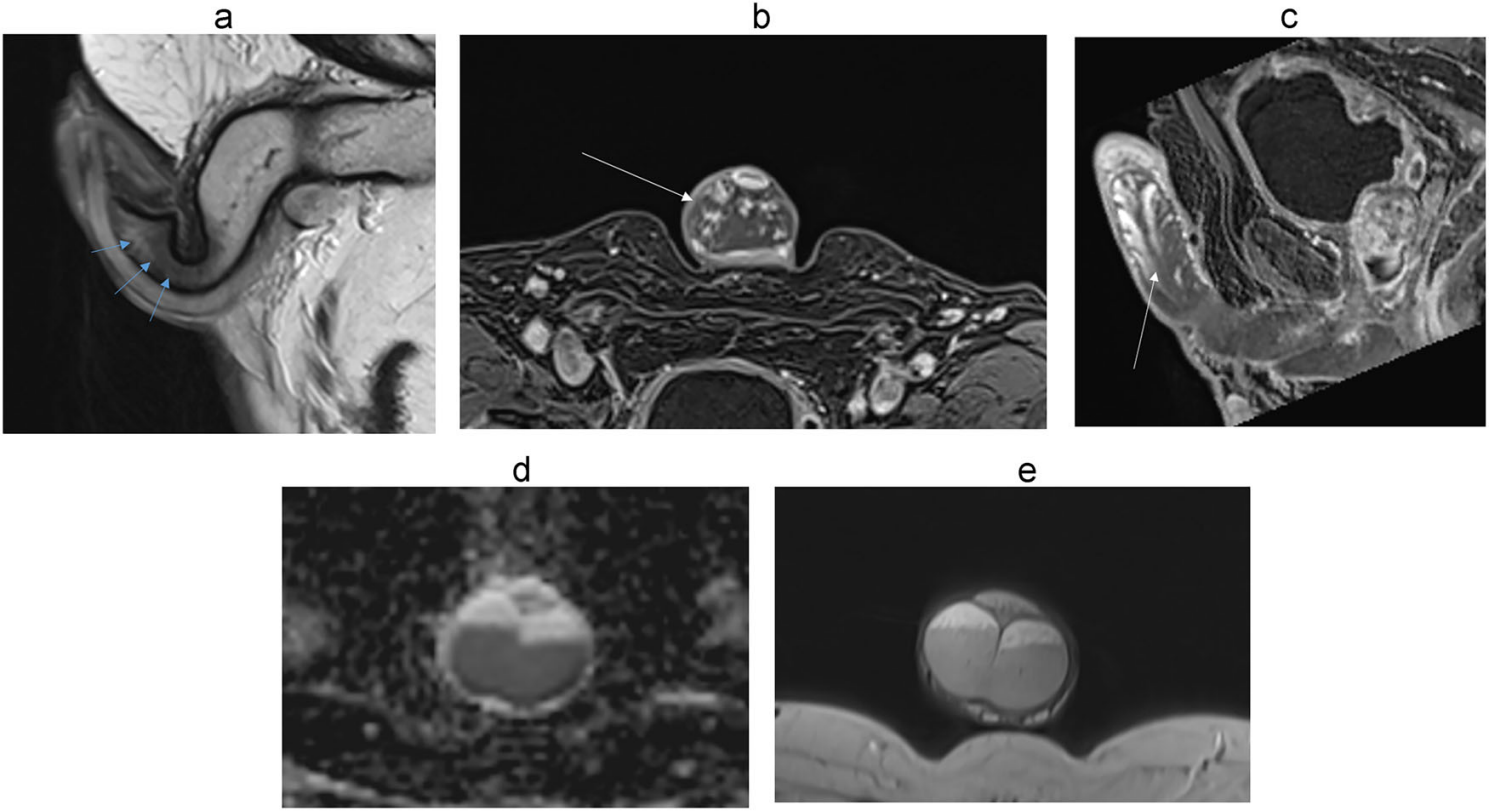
Common imaging pitfalls: T2 hyposignal areas in the flaccid penis (a), progressive enhancement of the corpora cavernosa in the axial plane (b) and sagittal plane (c), blood layering artifact on diffusion (d) and T2-weighted sequence (e)
